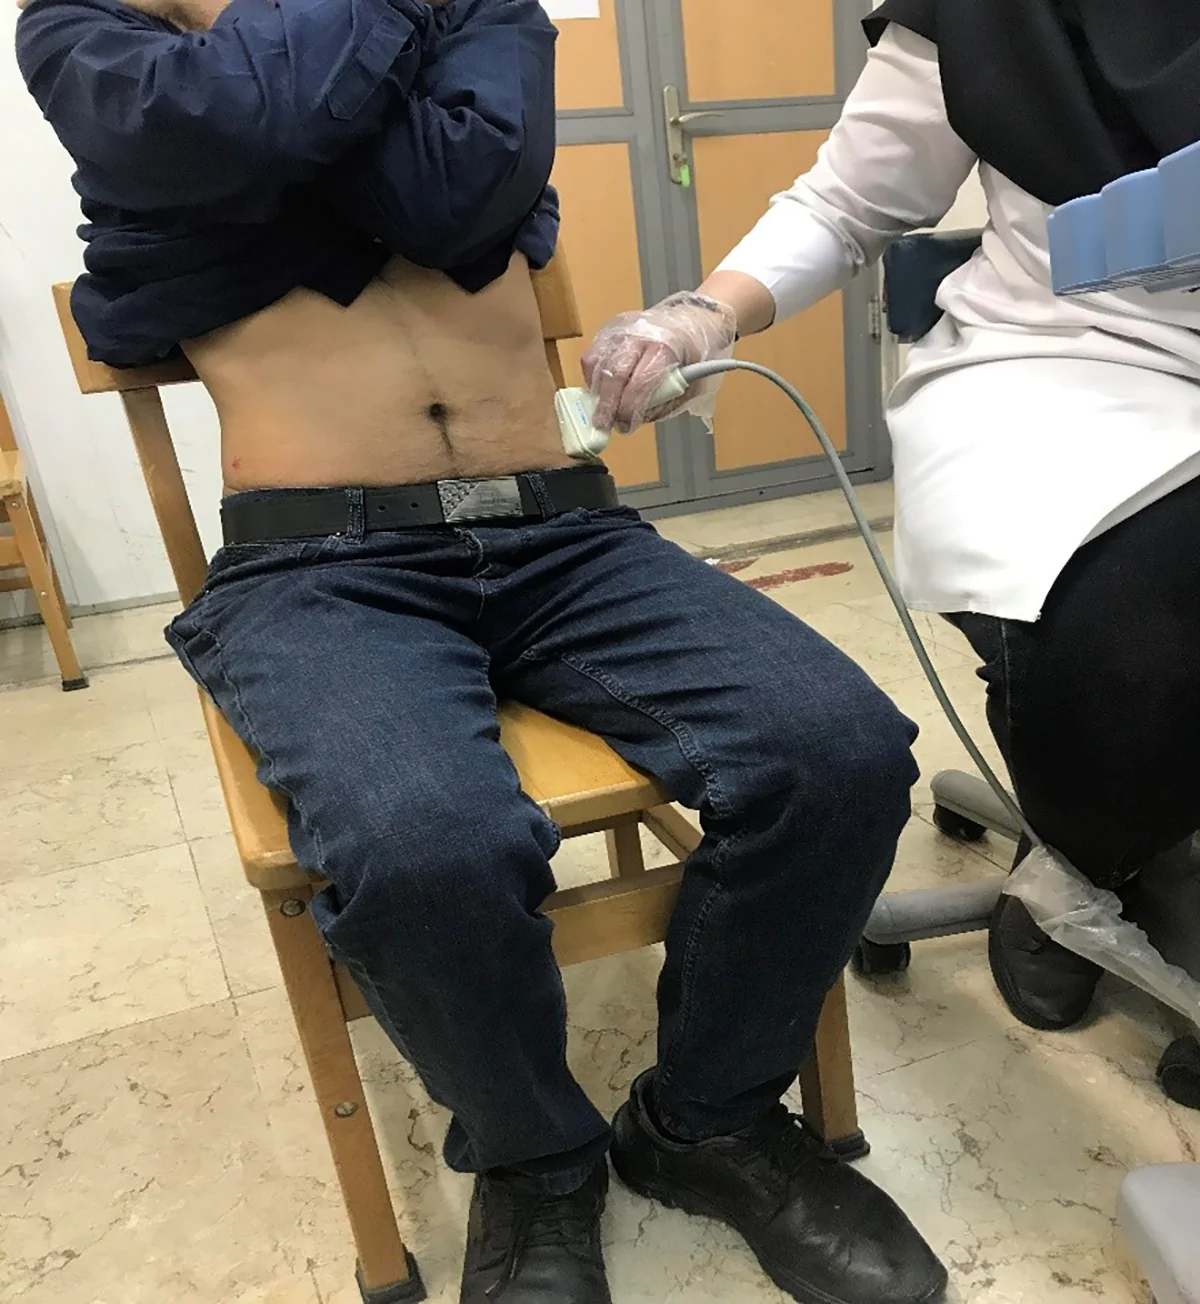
Ultrasound image showing abdominal muscle thickness in the sitting position. Created by the authors. Published under CC BY 4.0.
